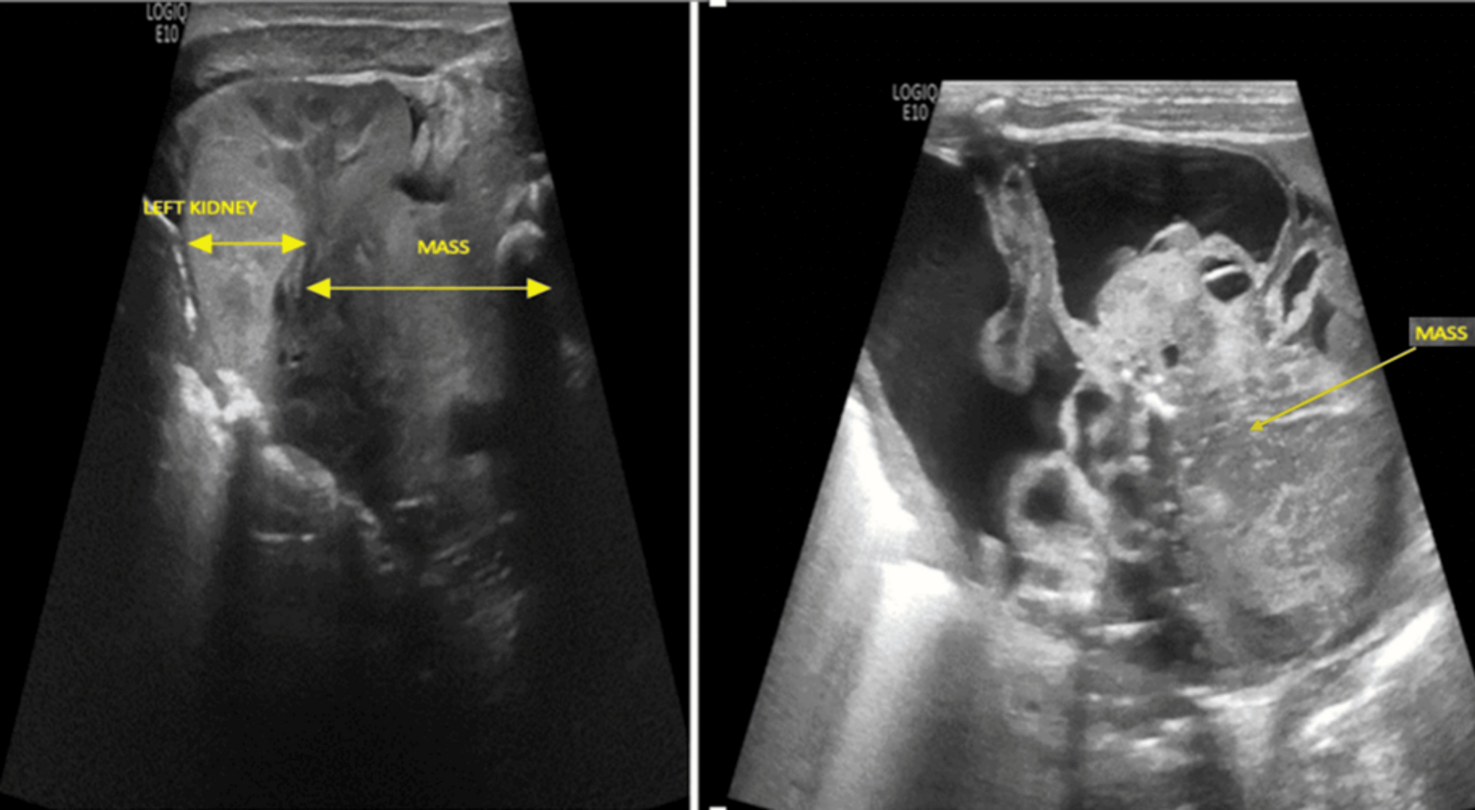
(Left, right) Abdominal ultrasound images revealing a large mass located above the left kidney, comprising multiple cystic and solid components.
